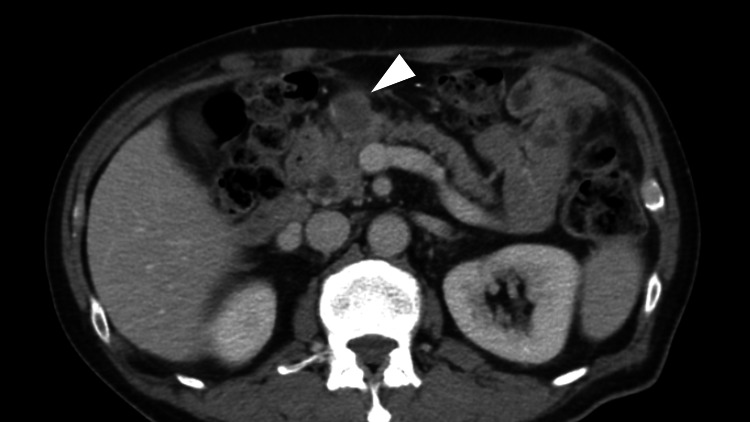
Axial abdominal contrast-enhanced computed tomography six months after laparoscopic distal gastrectomy An enlarged lymph node (arrowhead) is observed in front of the pancreas.
